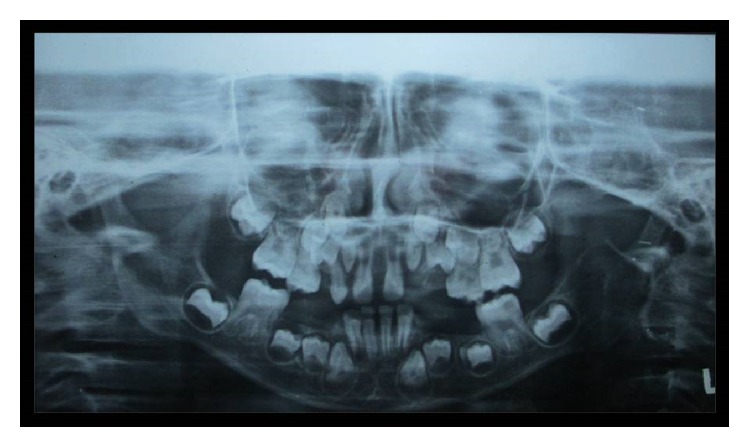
Panoramic radiograph showing lack of primary dentition tooth buds.
